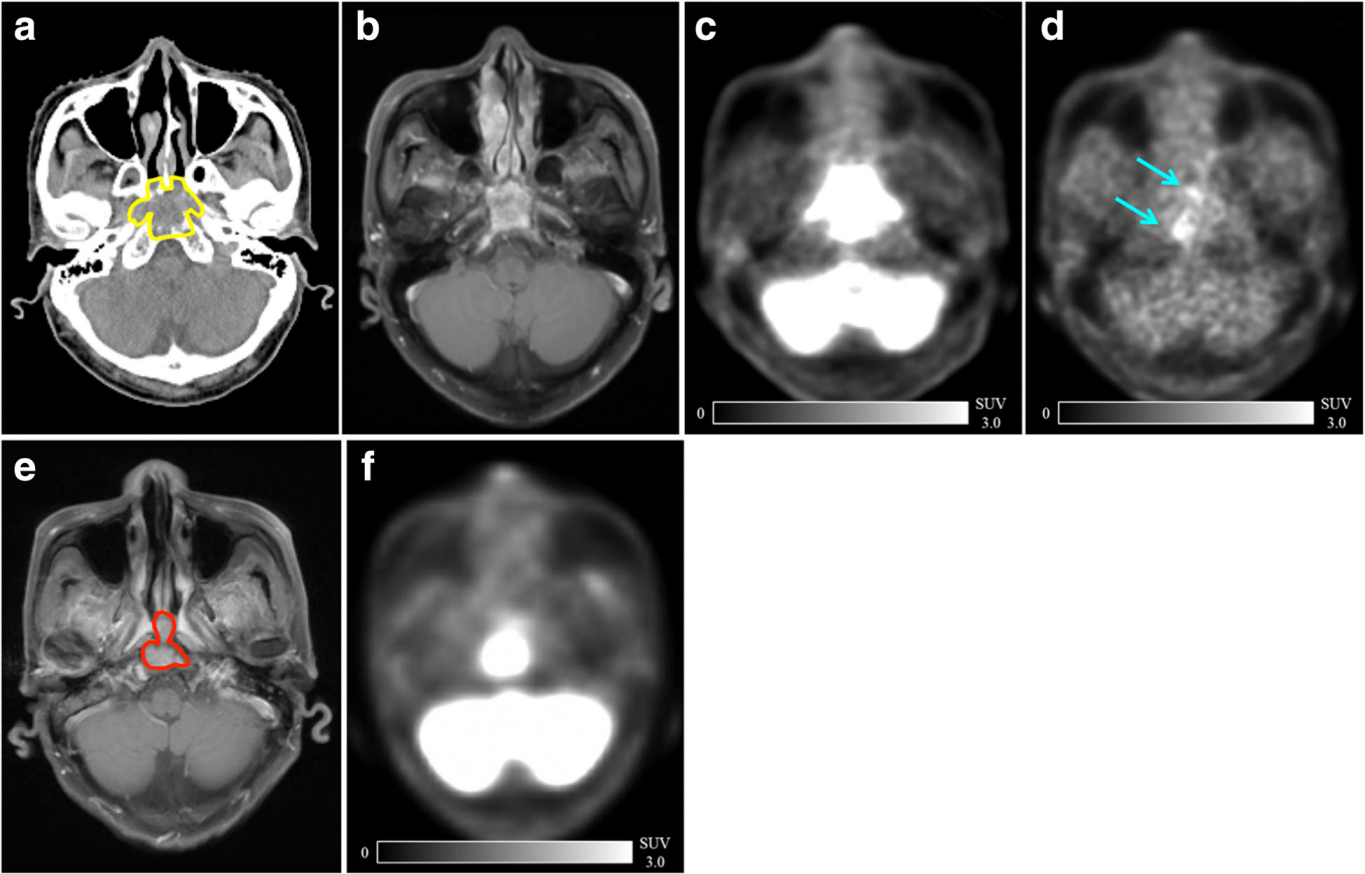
Representative cases. CT (a), contrast-enhanced MRI (b), FDG-PET (c) and FMISO-PET (d) were performed before radiation therapy. The CT showed nasopharyngeal mucosal disease and invasion to the clivus (yellow line). The high-uptake area of FMISO was distinguished at the central part of mucosal region and slightly to the right side of the clivus (cyan arrow). Five months after chemoradiation, this patient experienced local relapse. Contrast-enhanced MRI (e) and FDG-PET (f) showed recurrent tumor (red line). The relapse site was central to the nasopharyngeal mucosa and clivus and of a similar position with the pretreatment FMISO uptake area. *Abbreviations: CT*, computed tomography; *FDG-PET*, ^18^F- fluorodeoxyglucose positron emission tomography; *FMISO-PET*, ^18^F–fluoromisonidazole positron emission tomography
